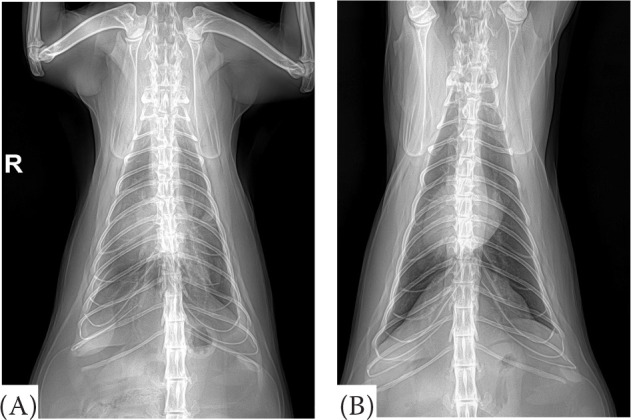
Ventrodorsal thoracic radiographs before (A) and after (B) treatment (A) Initial radiograph shows pulmonary oedema and pleural effusion. (B) A follow-up radiograph taken 2 days after treatment shows improvement in pulmonary oedema and pleural effusion
